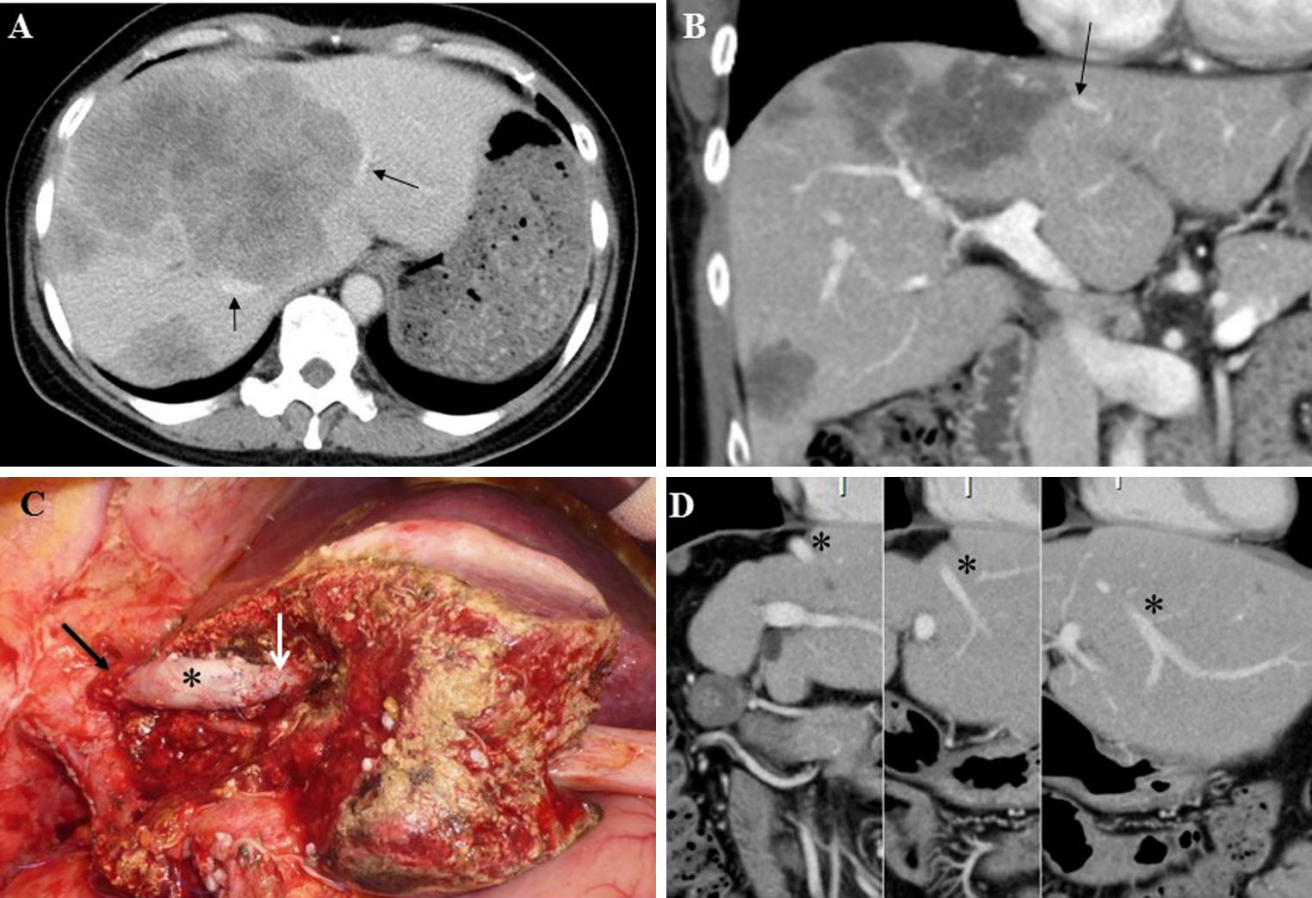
Extended right hepatectomy with left hepatic vein (LHV) reconstruction using interposition of an autologous external iliac vein graft (case 7 in Table 1). Initially, the patient’s tumor condition was diagnosed as unresectable because of massive multiple tumors predominantly in the right liver, with invasion of the right hepatic vein (RHV) and the trunk of the middle hepatic vein (MHV) and LHV (*arrows* on a). After seven courses of mFOLFOX6/panitumumab, the tumors shrank significantly, and the trunk of the MHV + LHV still was involved by the tumor (*arrow* on b). An external iliac vein interposition graft (*asterisk*) 5 cm long has been anastomosed between the distal stump of the LHV (*white arrow*) and the interior vena cava (IVC) orifice of the MHV + LHV trunk (*black arrow* on c). A computed tomography (CT) scan 12 months after hepatectomy shows the reconstructed LHV to be patent (*asterisk* on d). At this writing, the patient is alive without recurrence 24 months after the hepatectomy
